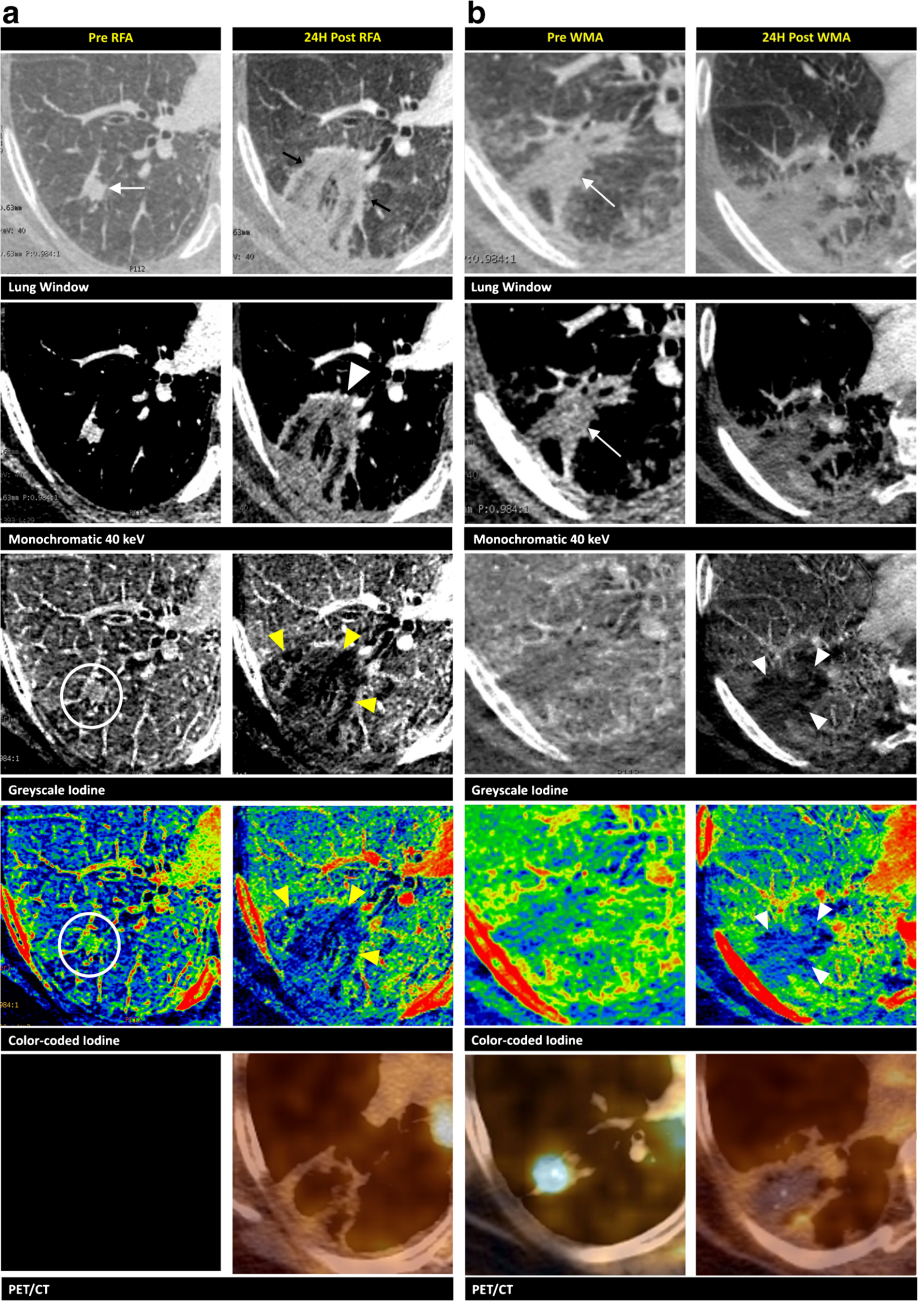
Pulmonary metastases. A 77-year-old female, with a known melanoma, undergoes a PET/CT that shows a hypermetabolic nodule in the right upper lobe (not in our possession). The biopsy is non-conclusive. The therapeutic option is RF ablation. a Imaging is performed before (first column) and 24 h after (second column) RF ablation. The 70-keV lung window shows a focal nodule of 11 mm (*white arrow*) in proximity of a blood vessel. This nodule is hyperdense on the synthesised monochromatic 40-keV images and shows iodine content on the greyscale- and colour-coded iodine maps (*circles*). Destructive lung parenchyma is seen after the ablation as an irregular hyperdensity on the lung window (*black arrows*). The synthesised monochromatic 40-keV reconstructions show very heterogeneous densities in this region. The vessel on the anterior side is still intact (*white arrowhead*). On the iodine maps, we clearly observe the absence of iodine uptake (*yellow arrowheads*). PET/CT shows no activity in this region. b Imaging is performed before (first column) and 24 h after (second column) microwave ablation (MWA). After 6 months, a well-circumscribed consolidation is seen on the 70-keV lung window and synthesised monochromatic 40-keV image (*arrows*). The greyscale- and colour-coded iodine maps show no focal iodine uptake. This consolidation is hypermetabolic on PET/CT. As a result of the discrepancy between the morphologic and metabolic imaging methods, and the proximity of the blood vessel, which could lead to a heat-sink effect, the patient opts for further focal thermal treatment through microwave ablation. Twenty-four hours after ablation, a large heterogeneously shaped density is recognised on the lung window. The greyscale- and colour-coded iodine maps very clearly show the zone without internal contrast uptake (*arrowheads*) when compared to the 40-keV images. Again, a photopenic area is seen on the PET/CT image
